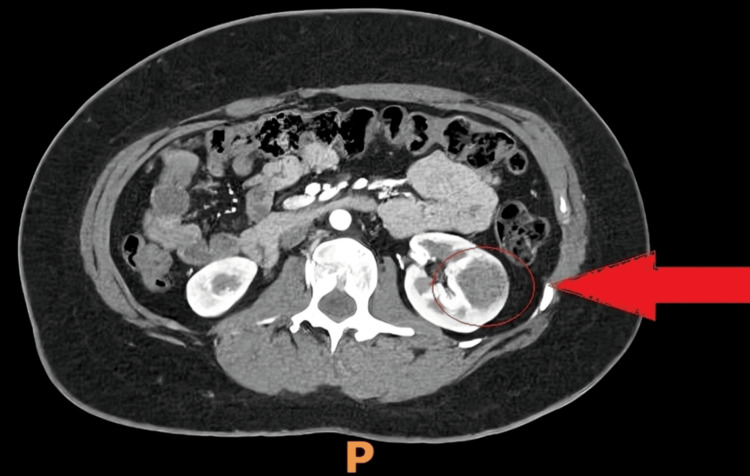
An axial view of CT thorax and abdomen with contrast showing a 2.8 cm left mid-pole renal lesion (red arrow and circle).
